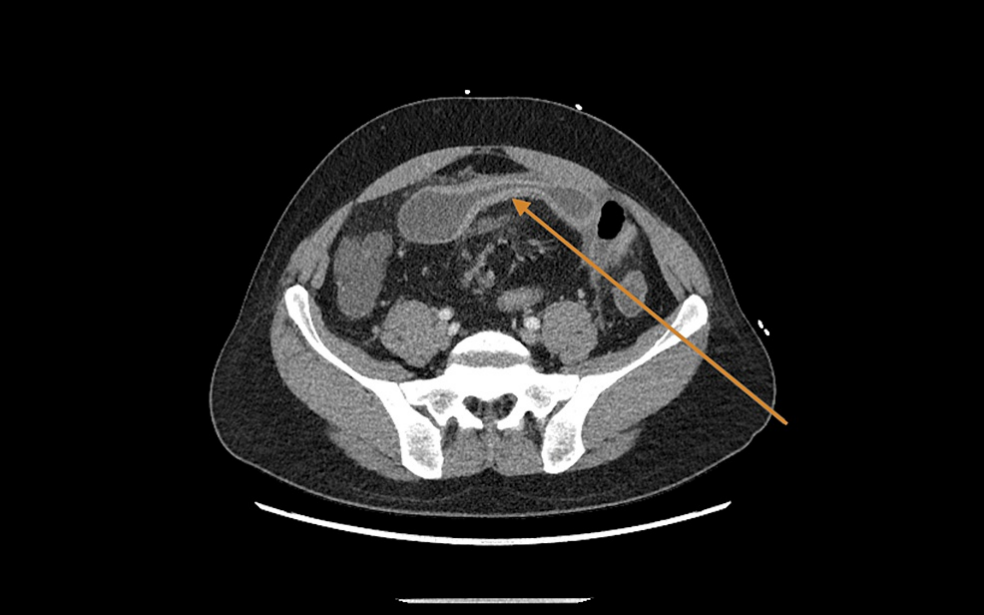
Computed tomography imaging shows an inflamed distal ileum (arrow) consistent with Crohn’s flare.
